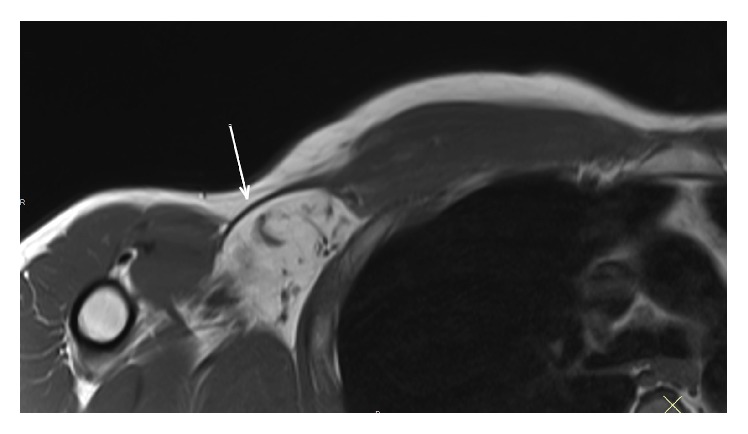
MRI axial T1 showing tendon retracted medial to the anterior chest wall and absence of any muscle fatty infiltration.
